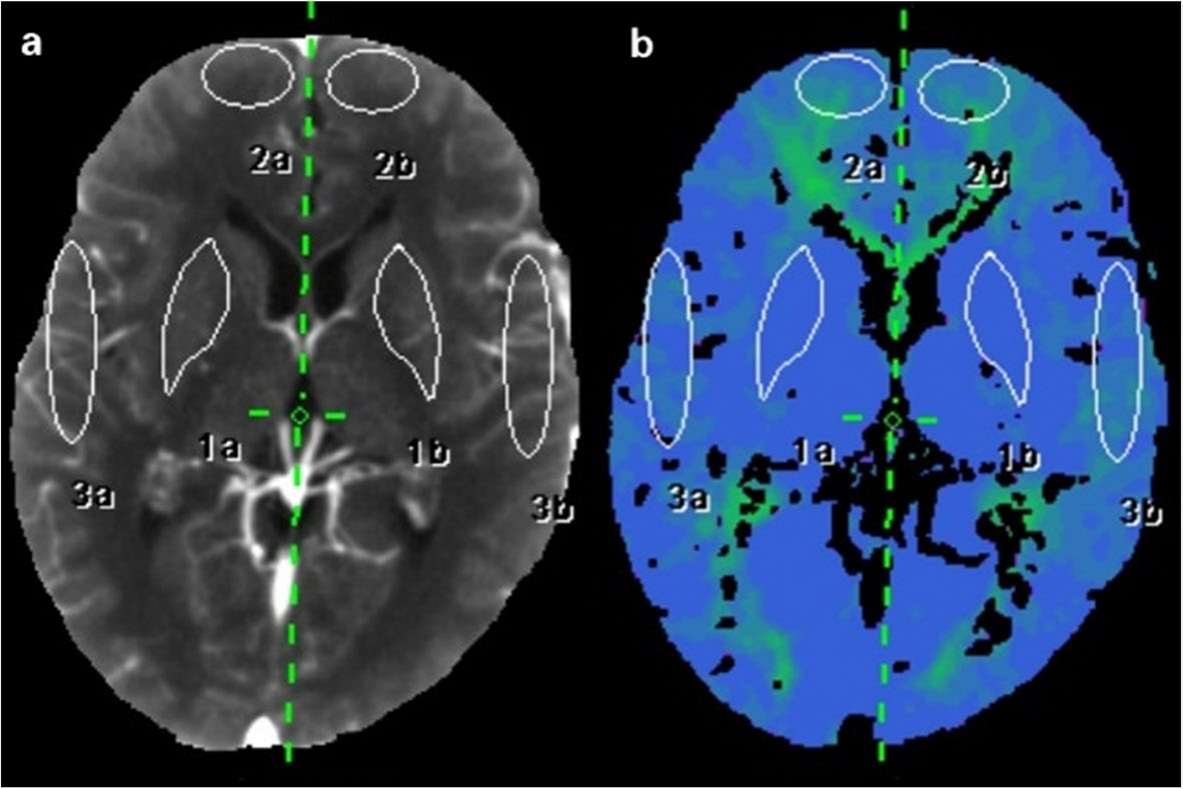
Example of regions of interest (ROIs) of the frontal lobe, operative area, and basal ganglia, drawn in the reference CT image (a) and time-to-peak map (b)
